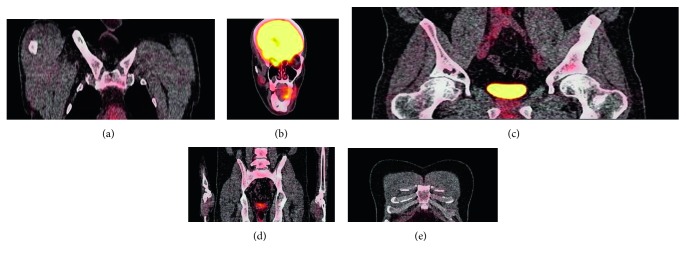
PET CT imaging without metabolic activity in stable lesions and no new lesions. (a) Right clavicle, (b) mandible region, (c) hip and pelvis, (d) pelvis and sacroiliac joint, and (e) sternum.
